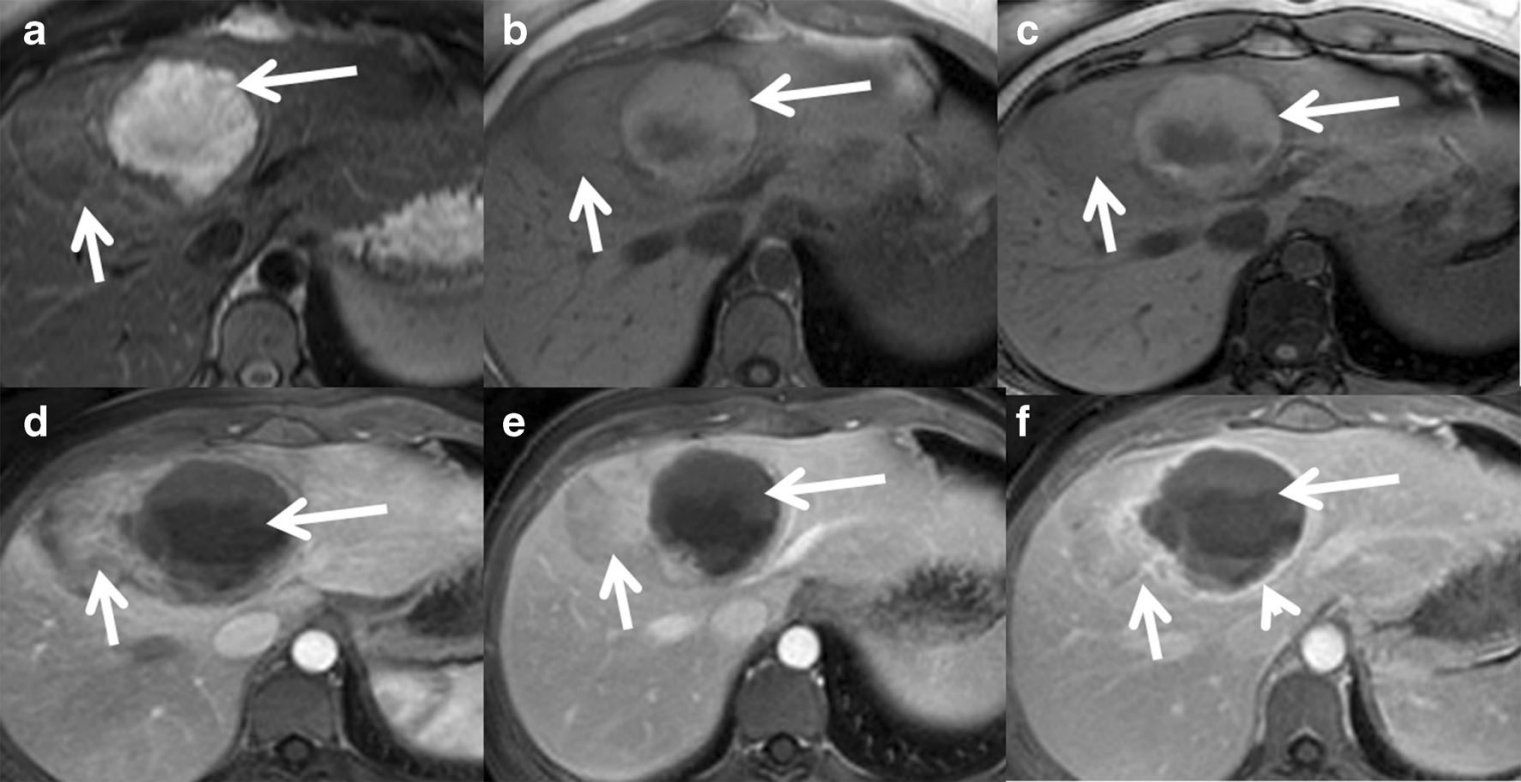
Hepatocellular adenoma with haemorrhage in a 40-year-old female on oral contraceptive pills. (a) Large heterogeneous lesion involving segment 8 and 4 of the liver showing a well-defined T2 hyperintense area along its medial aspect (*long arrow*) and T2 isointense area along its lateral aspect (*short arrow*). Axial T1 in-phase (b) and out-of-phase (c) MRI shows heterogeneous T1 hyperintensity in the medial portion (*long arrow*) without signal drop representing subacute haemorrhage. Axial post-contrast MRI shows heterogeneous mild-to-moderate enhancement of the lateral part of the lesion (*short arrow*) in the arterial phase (d) becoming isointense on venous (e) and delayed phases (f). The haemorrhagic component in the medial part of the lesion (*long arrow*) shows only peripheral capsular enhancement (*arrowhead*)
